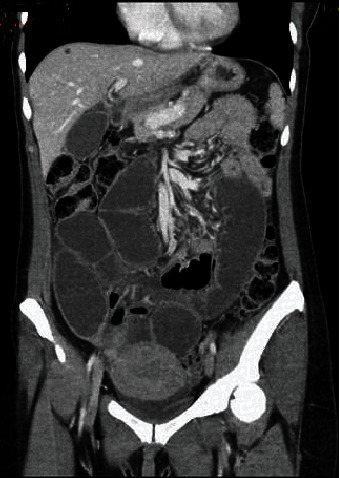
Abdominal computed tomographic scan showing dilated small bowel loops.
